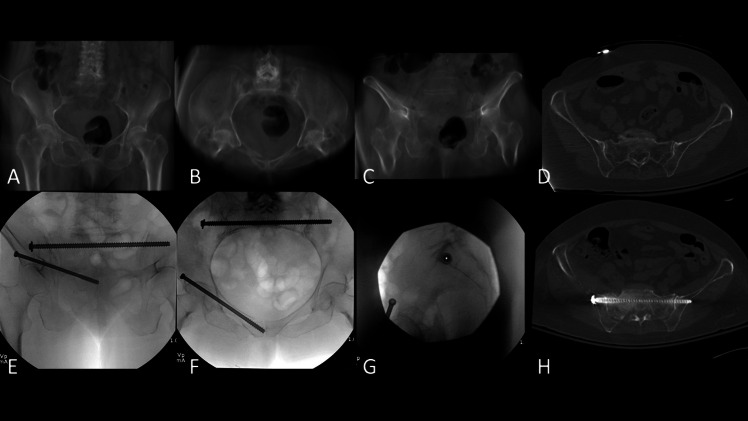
Computed tomography reconstructed (A) AP, (B) inlet, (C) outlet views and (D) axial CT image of 71-year-old woman a displaced LC1 fragility fracture of the pelvis. Intraoperative fluoroscopic (E) inlet, (F) outlet, and (G) lateral sacral views and postoperative (H) axial CT image demonstrating closed reduction internal fixation with an antegrade ramus screw and a fully-threaded transsacral screw
